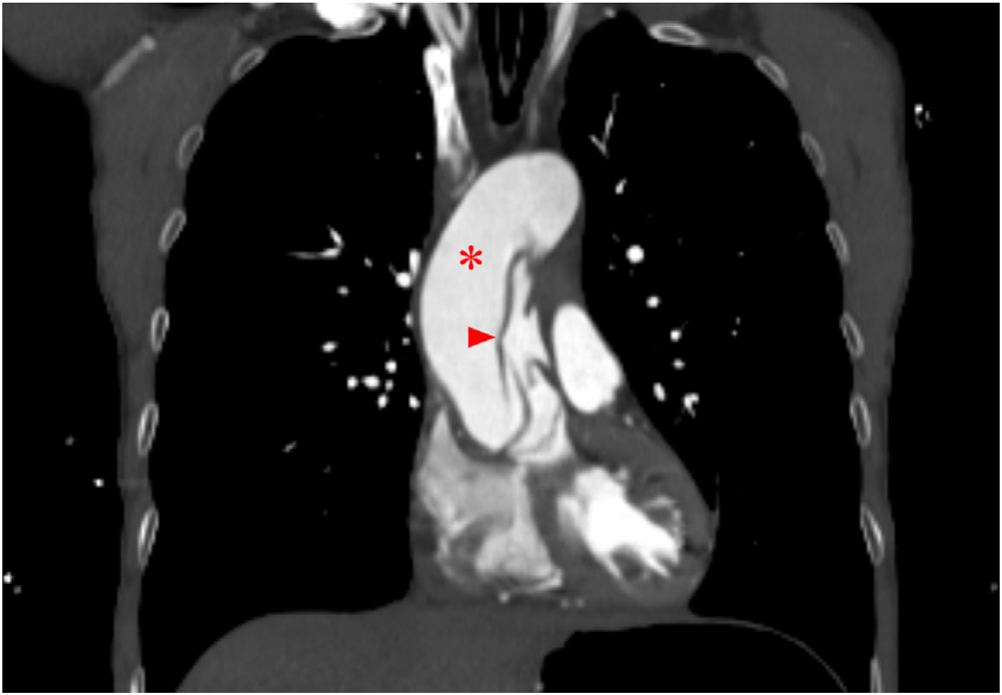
Coronal computed tomography angiography of the chest showing an ascending aorta (asterisk) with a dissection flap (arrowhead).
